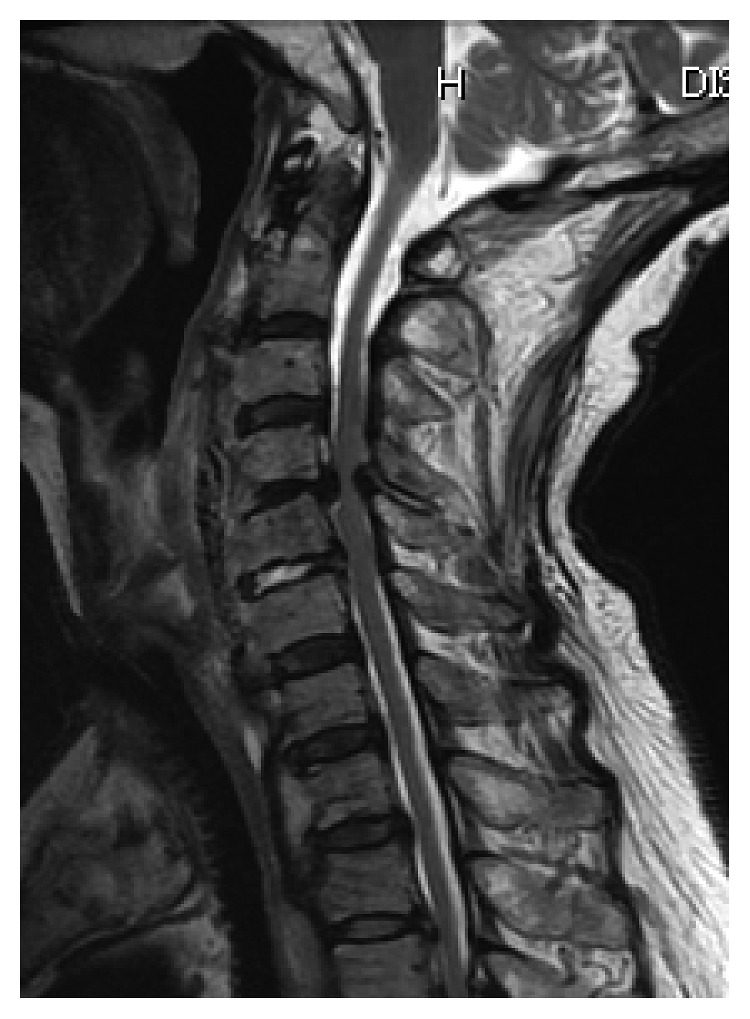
Magnetic resonance imaging after removal of OALL showing spinal canal stenosis due to ossification of the posterior longitudinal ligament at C4-5.
